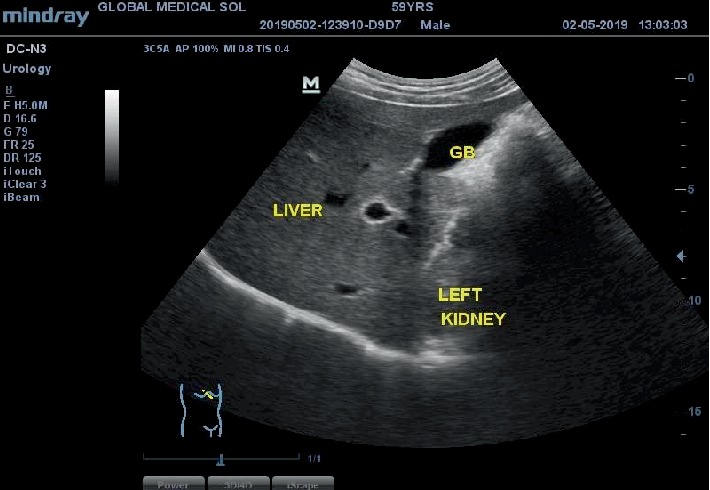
Ultrasound image of the epigastric region in longitudinal plane. This shows a left-sided liver and gallbladder instead of its normal right-sided anatomical position.
